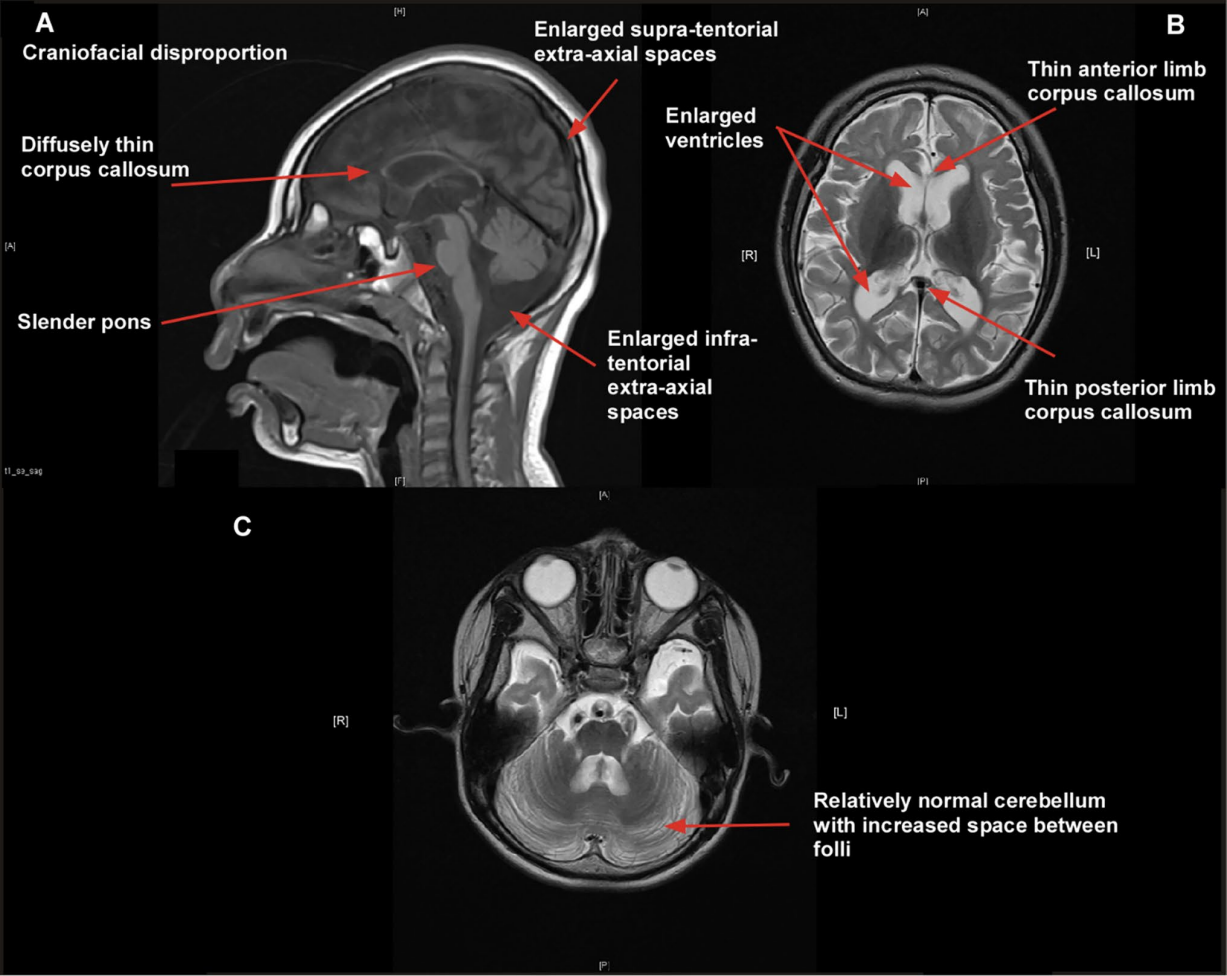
MRI of 4 year old boy with compound heterozygous pathogenic variants in *ASNS* (NM_183356.3:c. [866G > C]; [1010C > T]). A sagittal and B, C axial T2-weighted images showing small cranial vault with craniofacial dysmorphism in keeping with microcephaly, enlargement of the ventricles and the supratentorial and infratentorial extra-axial spaces in keeping with global atrophy in both the supratentorial and infratentorial compartments. Also noted are a diffusely thin corpus callosum, slender brainstem and a generalized, marked reduction of white matter volume. Head circumference was between − 1 and − 2 SD from the mean at birth but rapidly decelerated to be below − 5 SD from the mean by 20 months of age [200]
